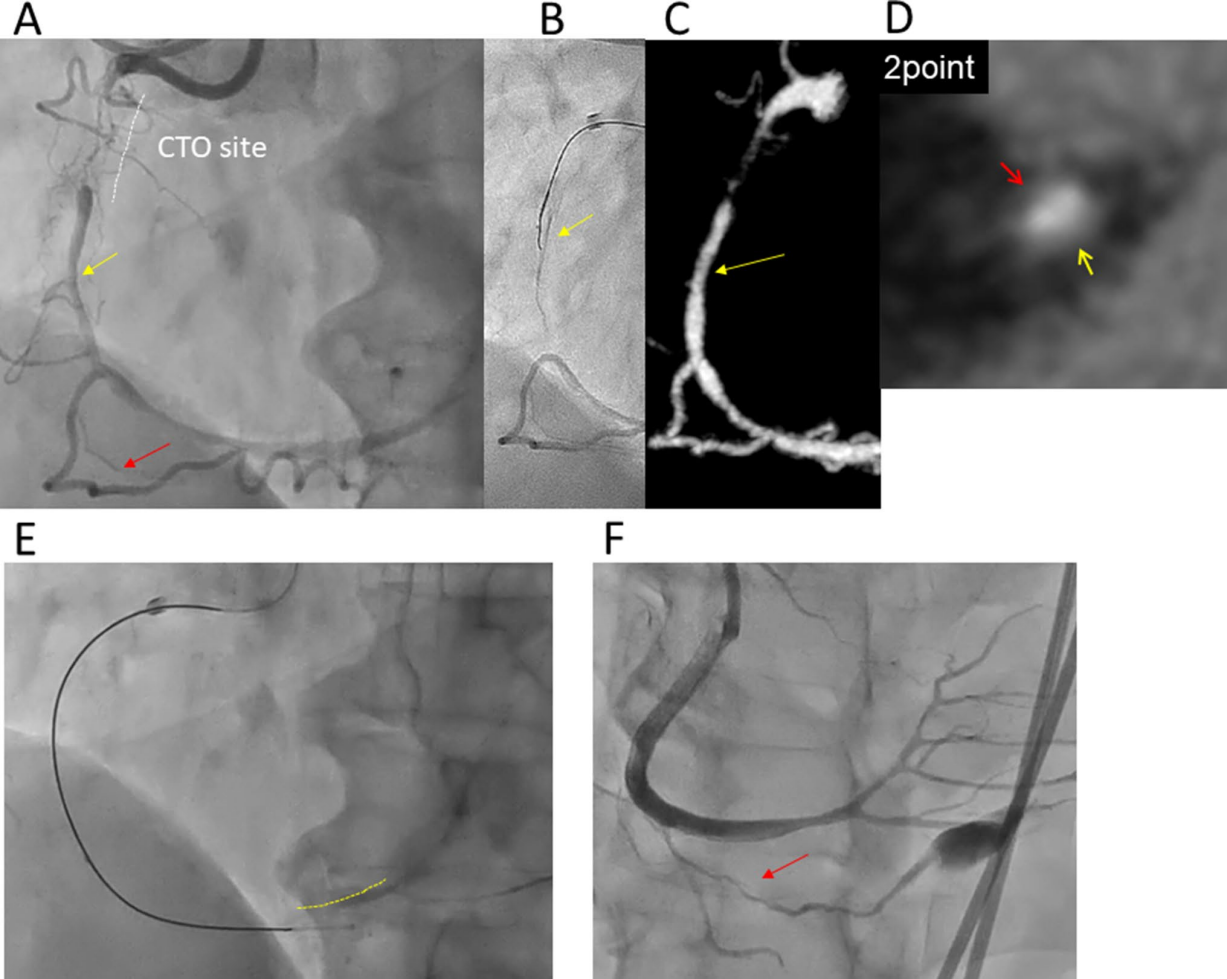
Case with coronary perforation with CrossBoss catheter. a Angiography showed chronic total occlusion (CTO) lesion at proximal right coronary artery (RCA). b Puncture with stingray wire and stingray balloon was performed at mid RCA (Yellow arrow), but it was failed. c Curved image of coronary computed tomography (CT). Yellow arrow indicated the 1^st^ puncture point according to the PCI angiographic image. d Cross sectional image at the puncture site and CT score was 2 point. Red arrow indicates the plaque at epicardial site and yellow arrow indicated the plaque at myocardial site. e After failure of the 1st puncture, CrossBoss catheter advanced to distal. However, the device was assumed to cross through outside of vessel because distal true lumen (Yellow dot line) visualized by contralateral angiography was completely far from the device. f Retrograde procedure was performed to bail out. The angiography after stent implantation showed coronary perforation because of CrossBoss cateter penetration into small branch of right ventricular branch (Red arrow). The case was treated without cardiac tamponade due to coil insertion into the branch
